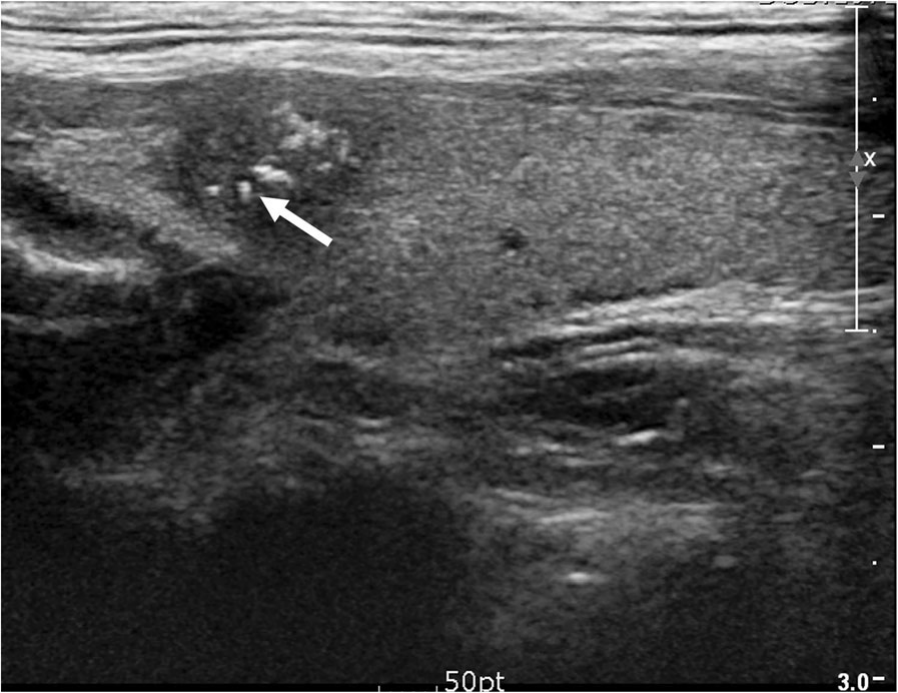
Echogenic foci associated with malignancy. 41-year-old male with thyroid nodule. Ultrasound image shows a 1.0 cm hypoechoic, solid nodule with spiculated margin. Multiple echogenic foci (Type 6) are present, including echogenic foci with comet-tail artifact (Type 2, arrow). Biopsy result was papillary carcinoma and was confirmed at surgery
